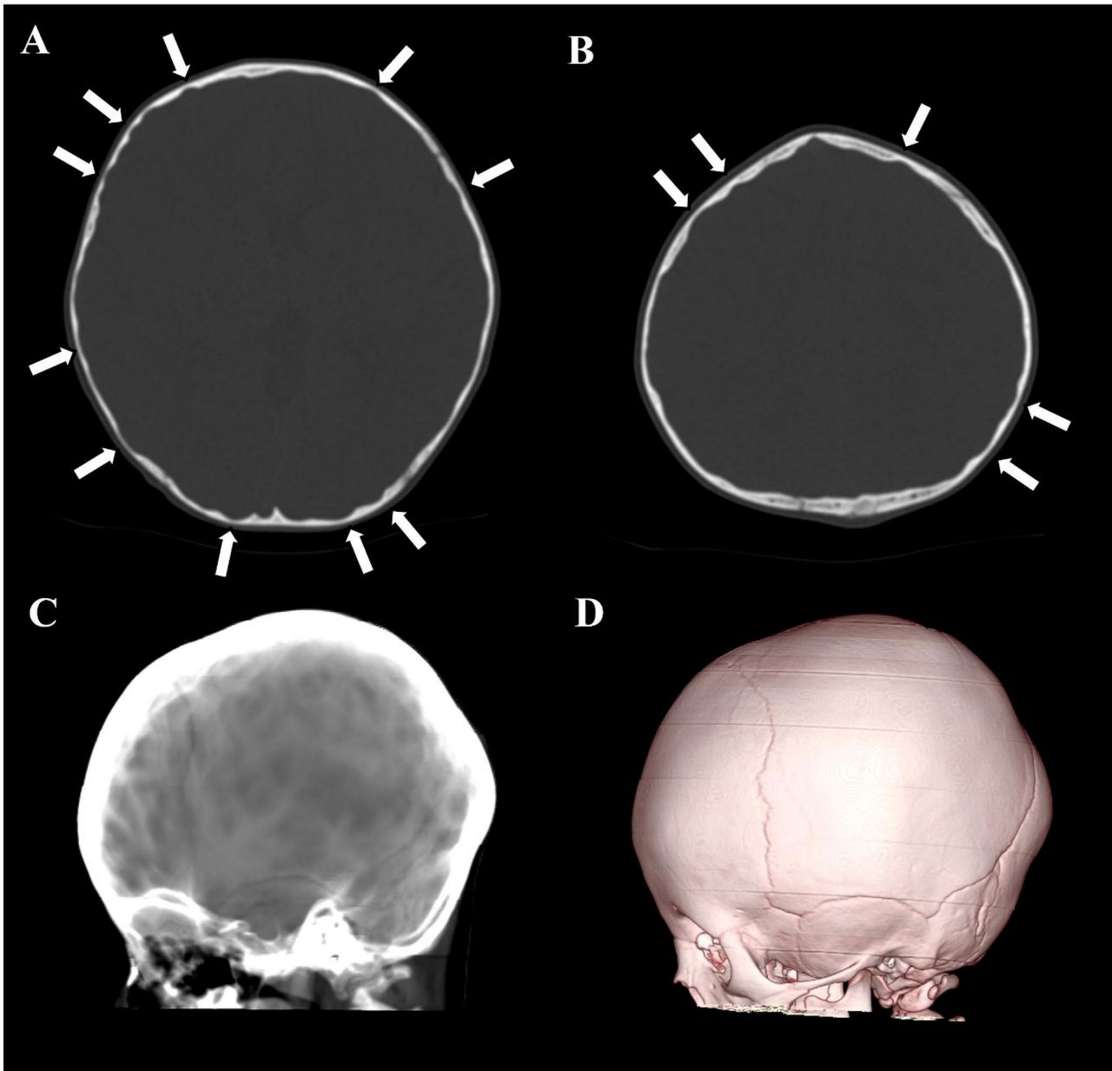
A 23-month-old boy with craniosynostosis demonstrating ICH. A, B Axial head CT images with bone window setting demonstrating multiple CT digital markings whole skull vault (arrows). C Ray-sum image demonstrating multiple focal hyperlucent areas due to CT digital markings entirely. D VR image, however, demonstrates no decompressions or holes, suggesting CT digital markings. Note that all cranial sutures are fused by craniosynostosis
